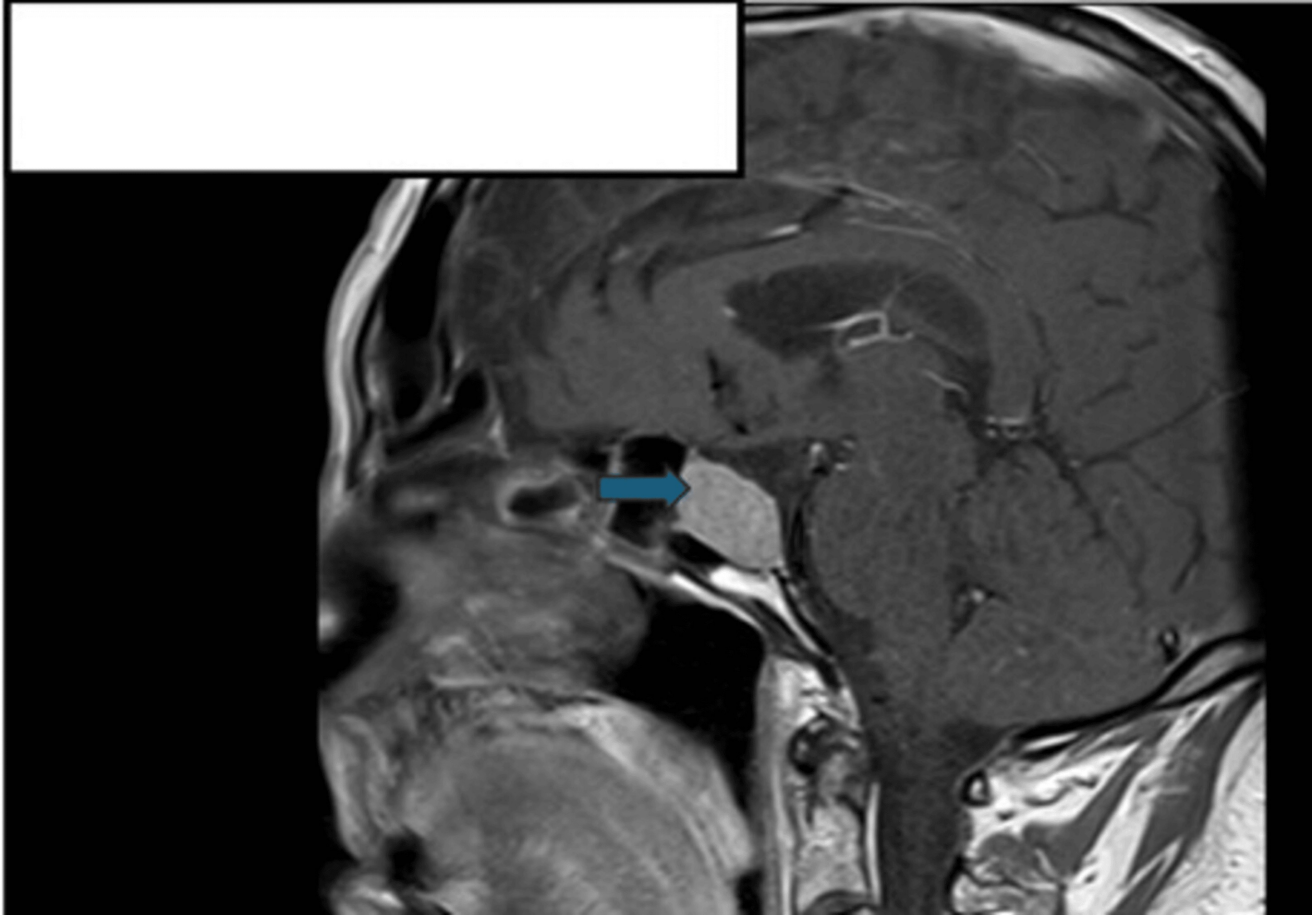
Sagittal section of a T1-weighted post-contrast MRI head scan performed eight years prior to this publication, revealing a possible macroadenoma (measuring 2.5 cm in maximum diameter) within the pituitary fossa. MRI: magnetic resonance imaging
